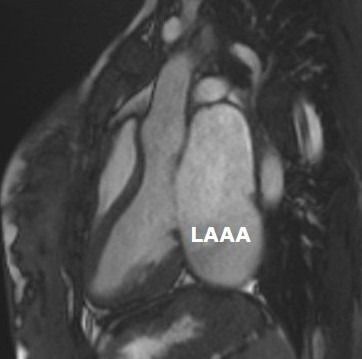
Cardiac MRI. Sagittal 3-chamber view from cardiac MRI
